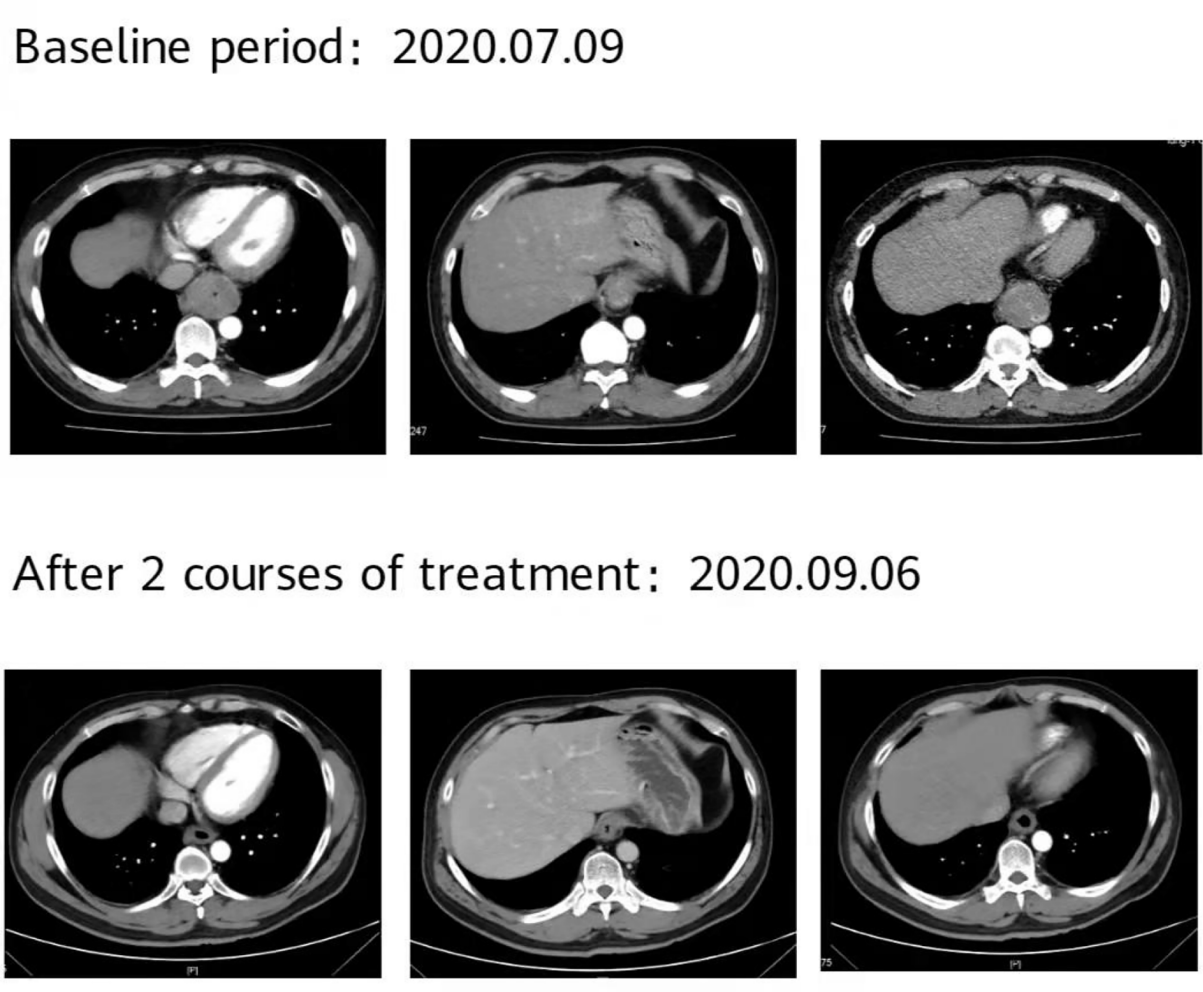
The patient’s imaging examination efficacy evaluation was based on the Response Evaluation Criteria in Solid Tumours 1.1. After 2 courses of treatment, re-examination of the second-phase enhanced CT of the lungs showed that the esophageal lesions were reduced compared with the baseline, and the curative effect was evaluated as partial remission (PR).
